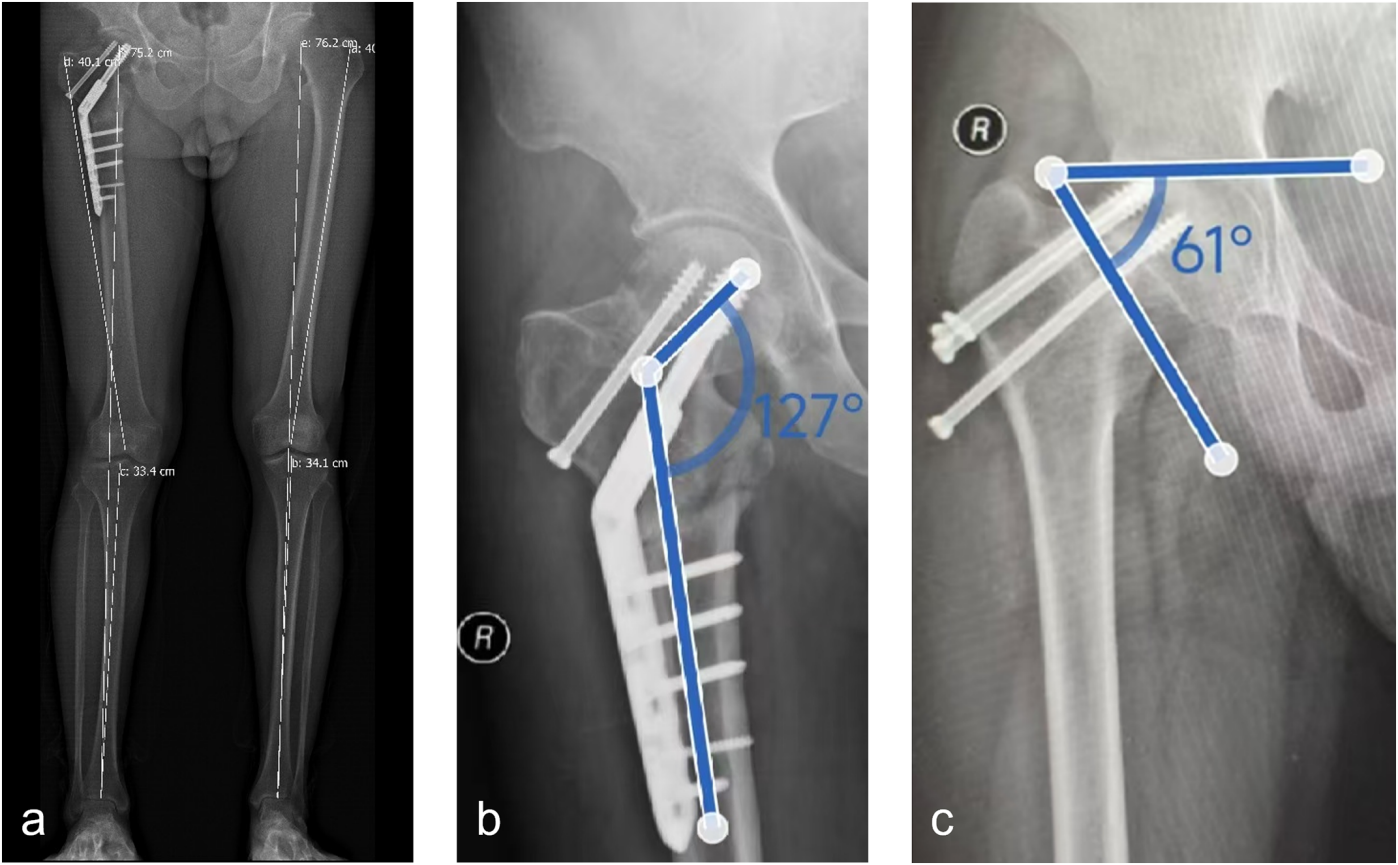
Test methods for the mean neck-shaft angle, pauwels angle, and limb length. (a) Limb length: Calculated as the sum of the femoral length (from the femoral head center to the distal condyle) and the tibial length (from the tibial plateau to the medial malleolus); (b) Neck-shaft angle: Measured on an anteroposterior radiograph of the hip as the angle formed by the intersection of the femoral shaft axis and the femoral neck axis; (c) Pauwels angle: Measured on an anteroposterior radiograph of the hip as the angle formed between the distal fracture line of the femoral neck and the line connecting the bilateral iliac crests.
